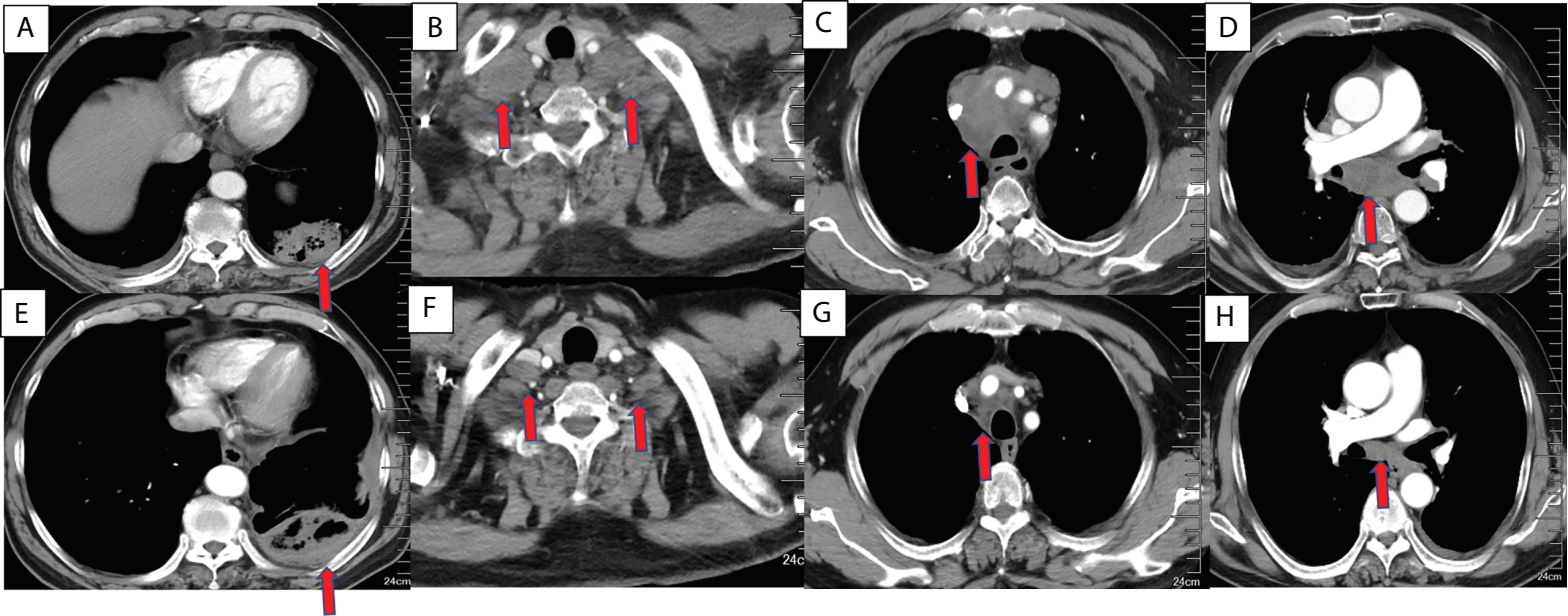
Chest CT findings of a 63-year-old male squamous cell lung cancer patient with a PD-L1 TPS of 1% who underwent MWA and camrelizumab monotherapy. (A–D) Baseline chest CT showing the primary tumor and lymph node metastases. (A) The primary tumor was located in the left lower lobe. (B) Bilateral supraclavicular lymph node metastases. (C, D) Mediastinal lymph node metastases in the 2R, 4R, 5, and 7 zones. (E–H) CT 2 months post-ablation and after four cycles of camrelizumab. (E). Complete ablation was achieved in the primary tumor. (F–H). Bilateral supraclavicular mediastinal lymph node metastases decreased dramatically. PD-L1, programmed death ligand-1; TPS, tumor proportion score; MWA, microwave ablation; CT, computed tomography.
